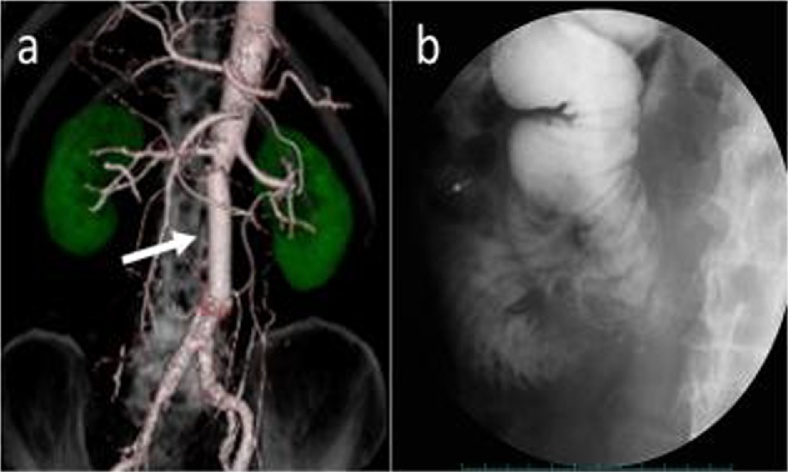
Abdominal computed tomography (CT) scan and duodenography. (a) Postoperative abdominal CT scan revealed that the I-shaped graft had good patency (white arrow). (b) Contrast media passed through the duodenojejunostomy without any problem.
